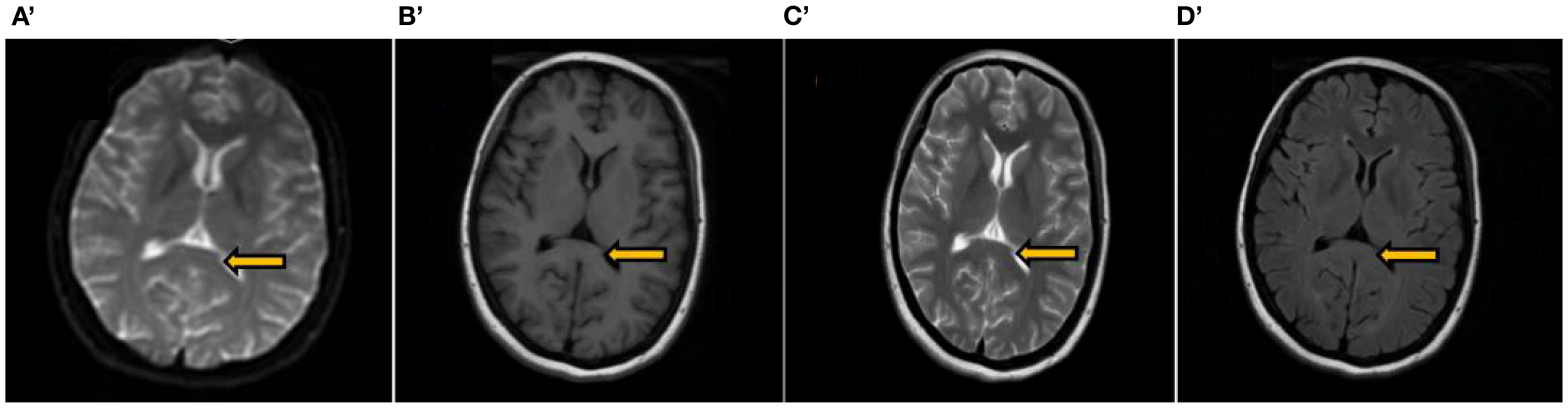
A review of the brain MRI conducted 2 months post-discharge. The images include the splenium of the corpus callosum (indicated by an arrow), along with DWI image (A’) T1-weighted image (B’) T2-weighted image (C’) and T2 Flair image (D’) all of which displayed no abnormal signals.
